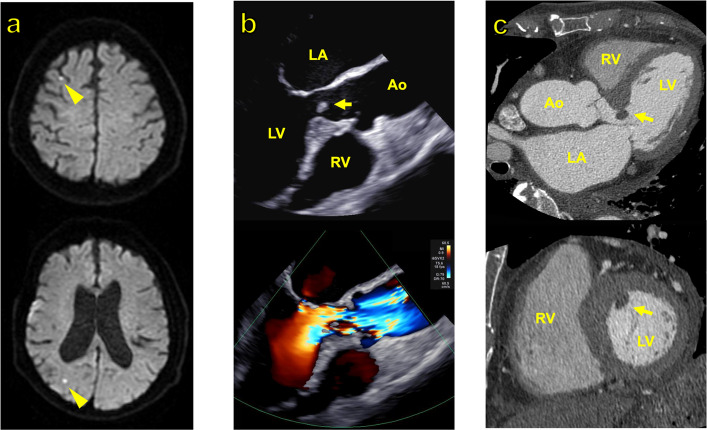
Preoperative imaging findings. A Brain MRI at presentation revealed multiple embolic lesions in both the subcortical and cortical areas of the cerebral hemispheres (arrowheads). B Preoperative transesophageal echocardiography (long-axis view at 135°) demonstrated a protrusion of the basal interventricular septum into the left ventricular cavity (sigmoid septum), with a mobile mass attached to it. Color Doppler imaging revealed a mosaic turbulent flow pattern in the LVOT, suggestive of systolic flow acceleration. C Preoperative contrast-enhanced cardiac CT (long-axis and short-axis views) of the left ventricle clearly visualized the sigmoid septum and the attached mass (arrow). Abbreviations: MRI, magnetic resonance imaging; LVOT, left ventricular outflow tract; CT, computed tomography; LA, left atrium; LV, left ventricle; RV, right ventricle; Ao, aorta
